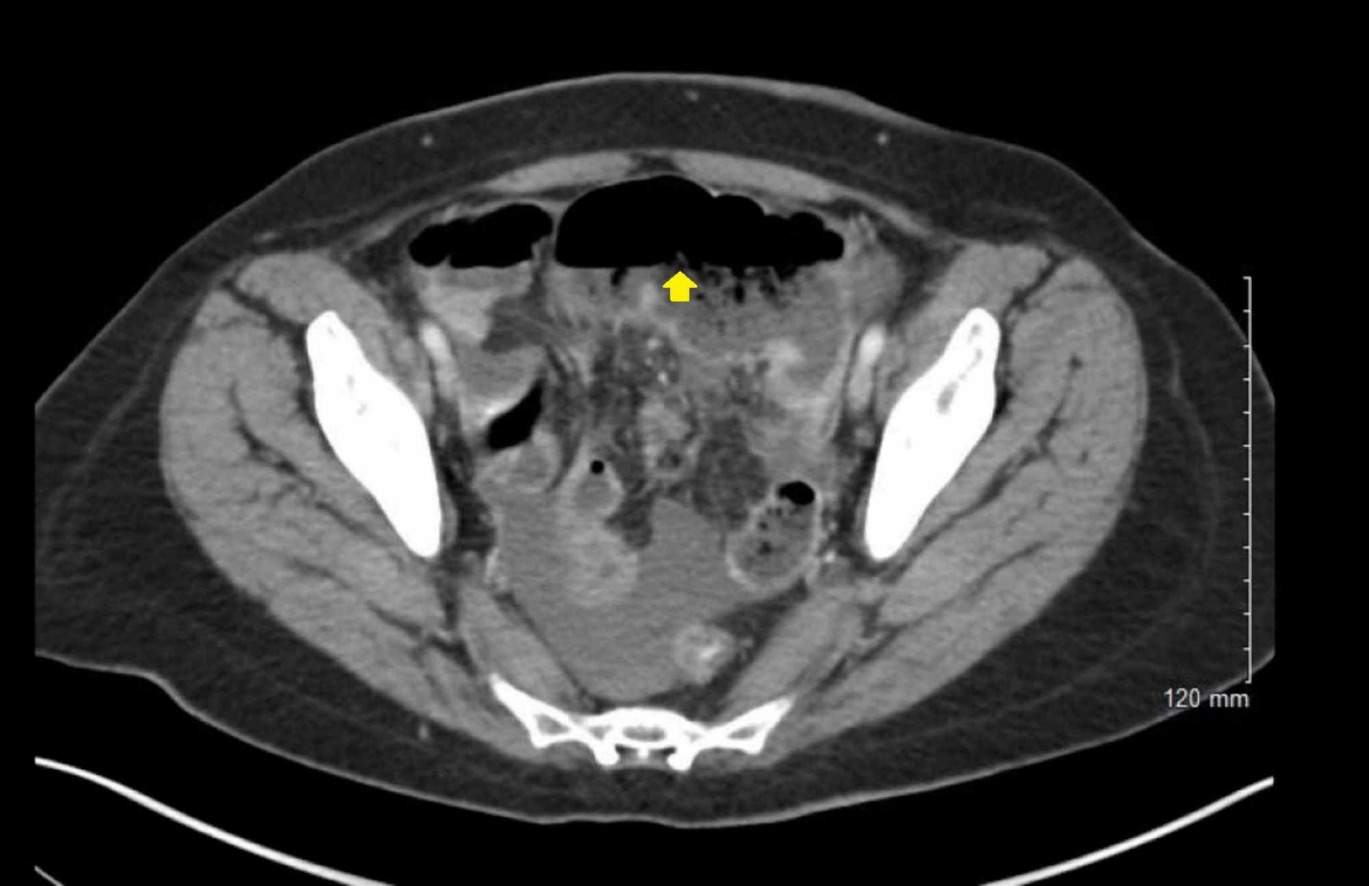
A CT scan of the abdomen (cross section) showing a fluid-filled loop of the small bowel suspicious for SBO. CT: Computed Tomography; SBO: Small Bowel Obstruction.
